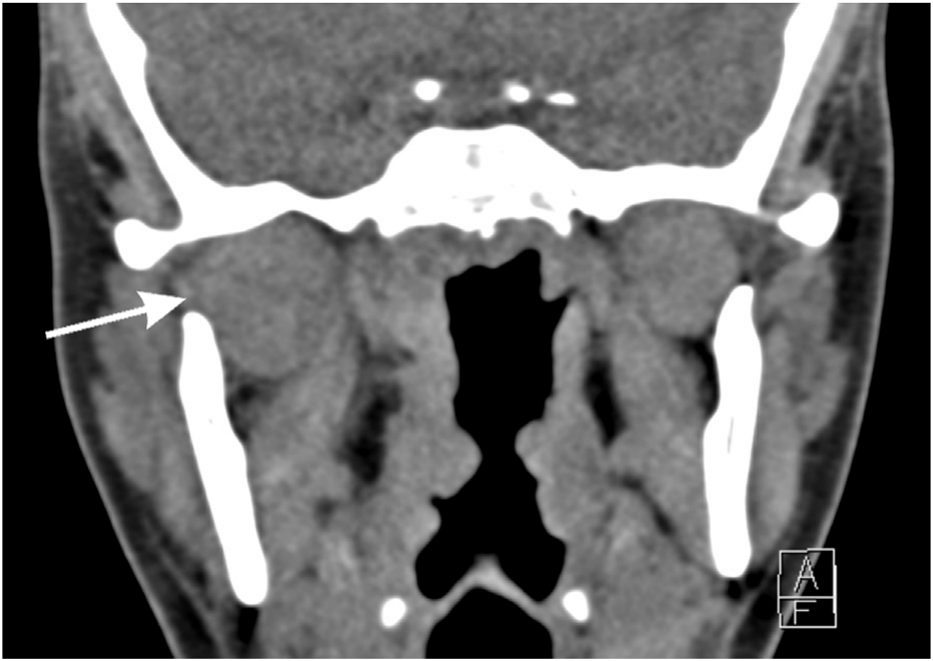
Computed tomography scanning of the head and neck. Coronal view showing enlargement of the right lateral pterygoid muscle and surrounding inflammation as well as fat infiltration, indicated with the arrow.
